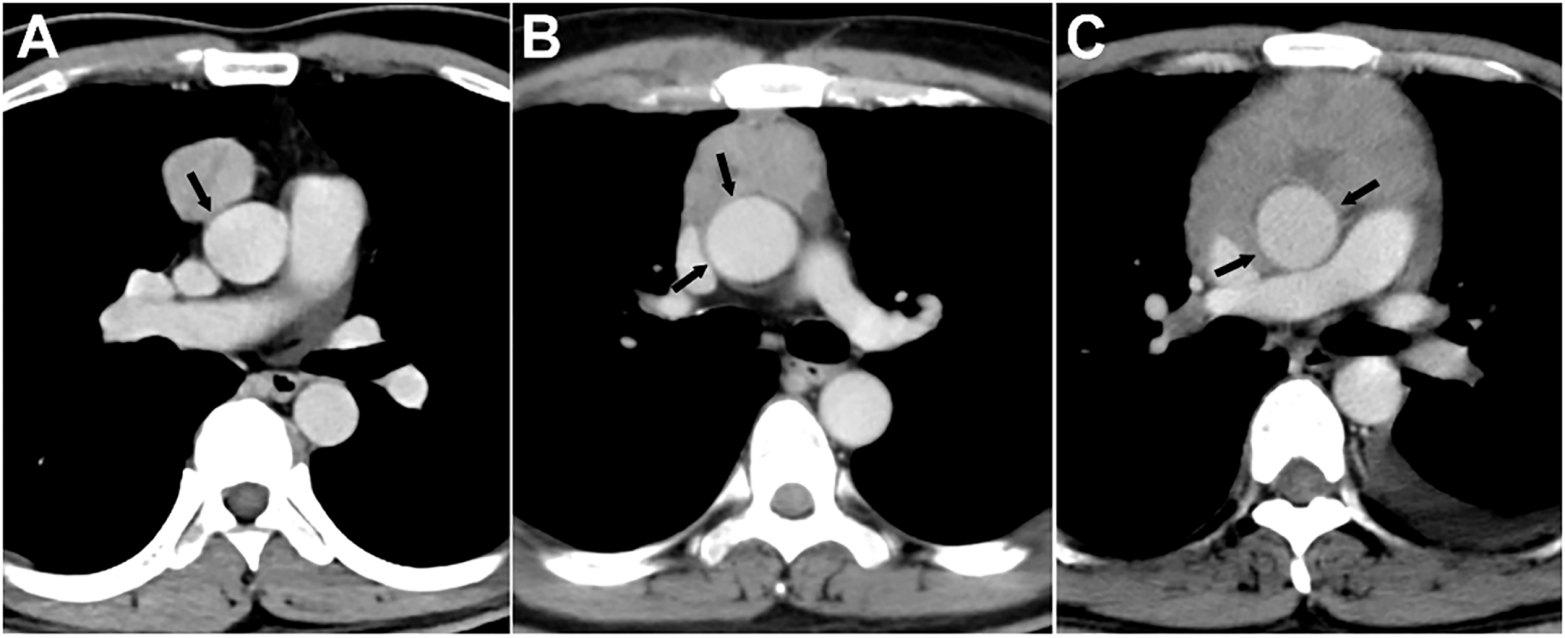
Grading of major mediastinal artery invasion on contrast-enhanced CT images. (A) Grade I, <25%. A 42-year-old man with type AB thymoma. Tumor abutment degree with adjacent ascending aorta is less than 25% (arrow) on axial venous phase enhanced CT image. (B) Grade II, 25%-49%. A 49-year-old man with thymic squamous cell carcinoma. Tumor abutment degree with adjacent ascending aorta is more than 25% but less than 50% (arrow) on axial venous phase enhanced CT image. (C) Grade III, ≥50%. A 40-year-old man with type B3 thymoma. Tumor abutment degree with adjacent ascending aorta is more than 50% (arrow) on axial venous phase enhanced CT image.
